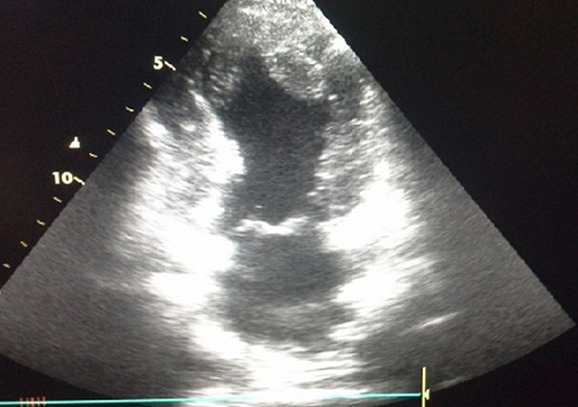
Transthoracic echocardiography: an apical two chamber view showing two enormous intraventricular apical thrombi
